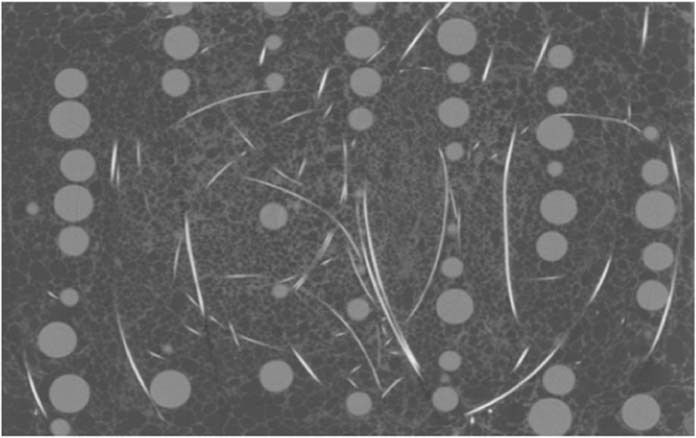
CT cross section of a sandwich material panel with NiCr wires reinforcing the hierarchical cellular structure.
